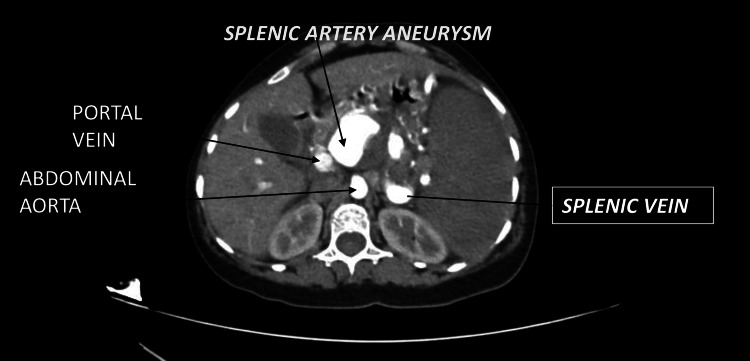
Contrast-enhanced CT Abdomen arterial phase showing splenic artery aneurysm with enhancement of the splenic vein and portal vein in the arterial phase suggestive of a fistula.
